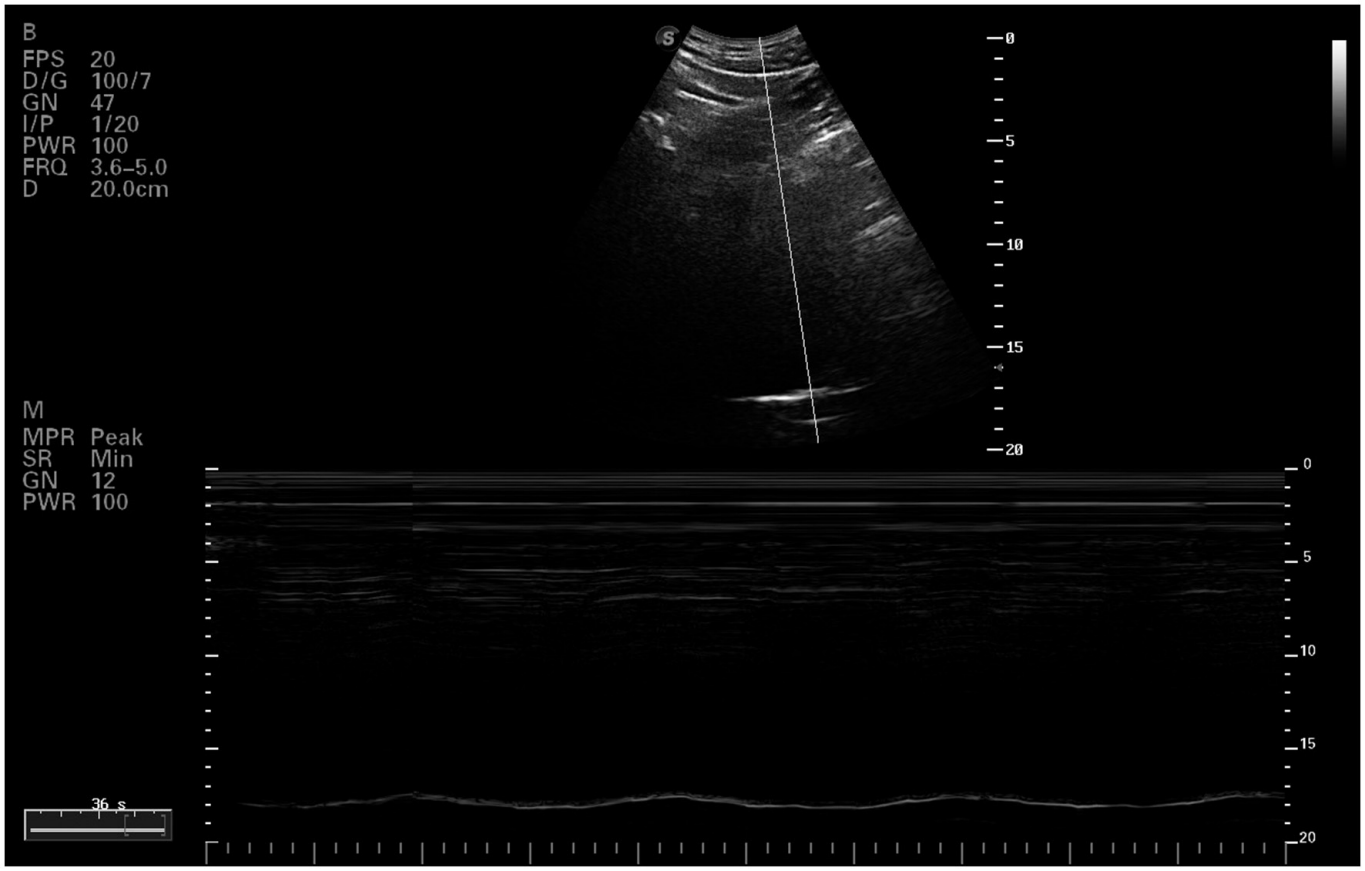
Ultrasound image of right diaphragmatic dome.
